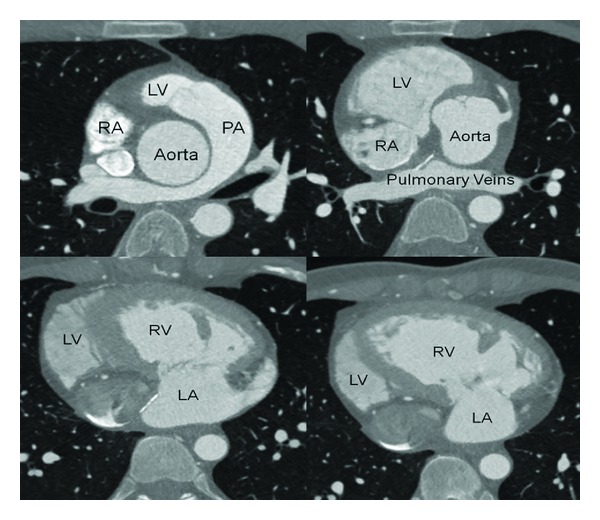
Anatomical confirmation of ccTGA on coronary CT (CTA).
